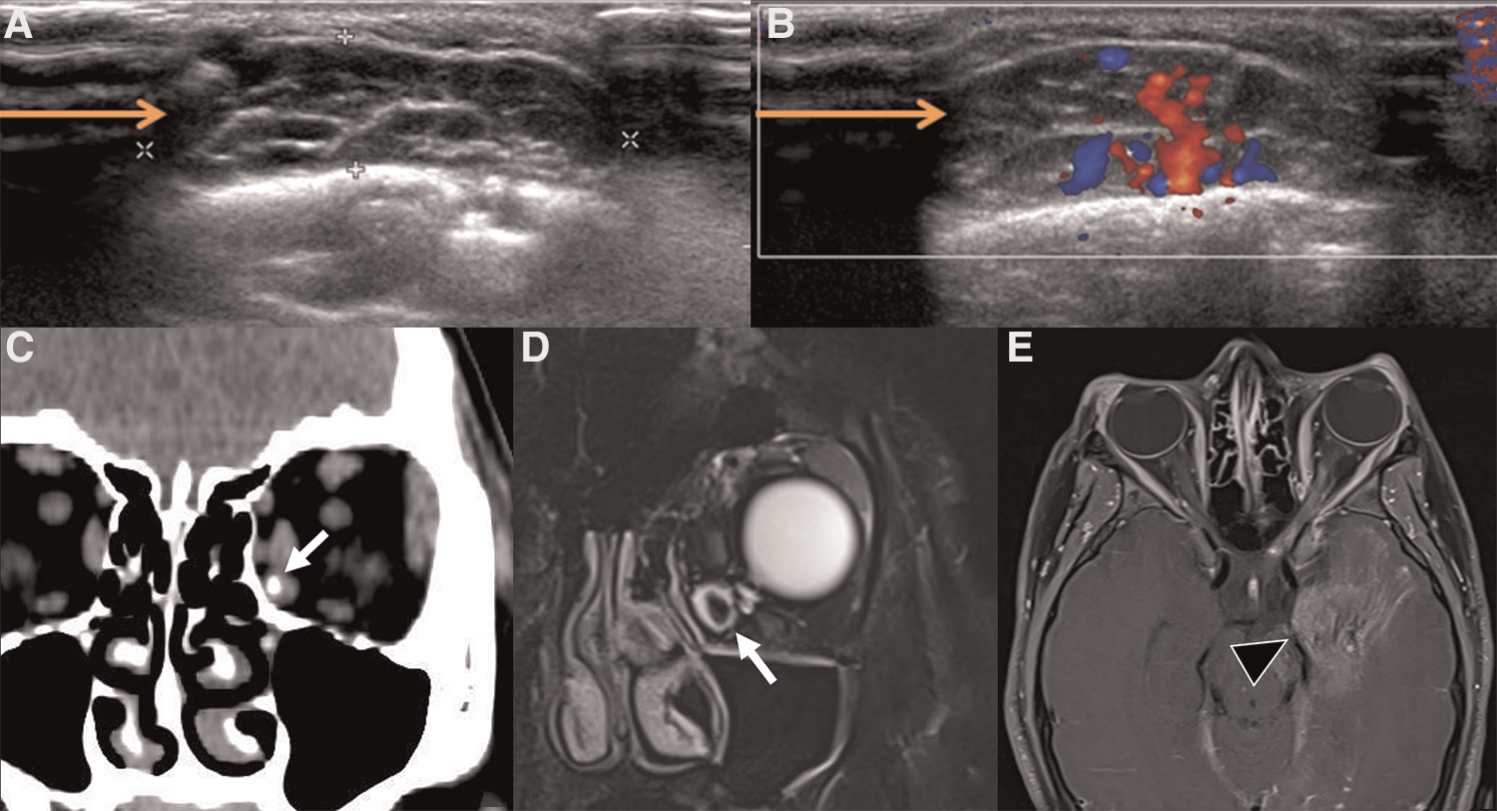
Imaging of venous malformations. (A,B) US of a venous malformation of the lid in a 7-year-old boy. B-mode US (A) shows a compressible, relatively well-marginated mass with a “spongiform appearance” consisting of multiple anechoic dilated venous spaces separated by hyperechoic septa; on color Doppler US during Valsalva maneuver (B) a slight increase in size and increased representation of color-Doppler signal (baseline not shown) can be appreciated. (C–E) Coronal CT (C), coronal fat-suppressed T2-weighted image (D) and axial post-contrast fat-suppressed T1-weighted images (E) of a venous malformation of the orbit in a 13-year-old girl. A phlebolith within dilated venous channels is clearly evident at CT and MRI (target sign, white arrows in C,D); axial post-contrast FS T1 weighted image also shows an associated diffuse hemispheric venous drainage anomaly (black arrowhead in E).
